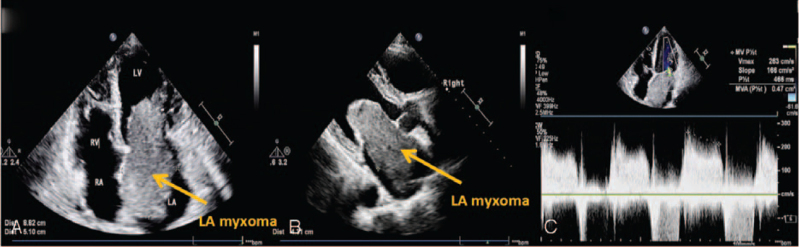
Echocardiographic imaging of LA myxoma in this patient. (A) Two-dimensional echocardiography revealed a mass of 88 mm in length and 51 mm in width (yellow arrow) in the left heart in the apical four chamber view. (B) Two-dimensional echocardiography showed a thick mass of 43 mm (yellow arrow) in the left heart in the apical two chamber view. (C) Pulse doppler imaging showed the accelerated forward flow of mitral valve. Mitral stenosis was noted. LA = Left atrial.
